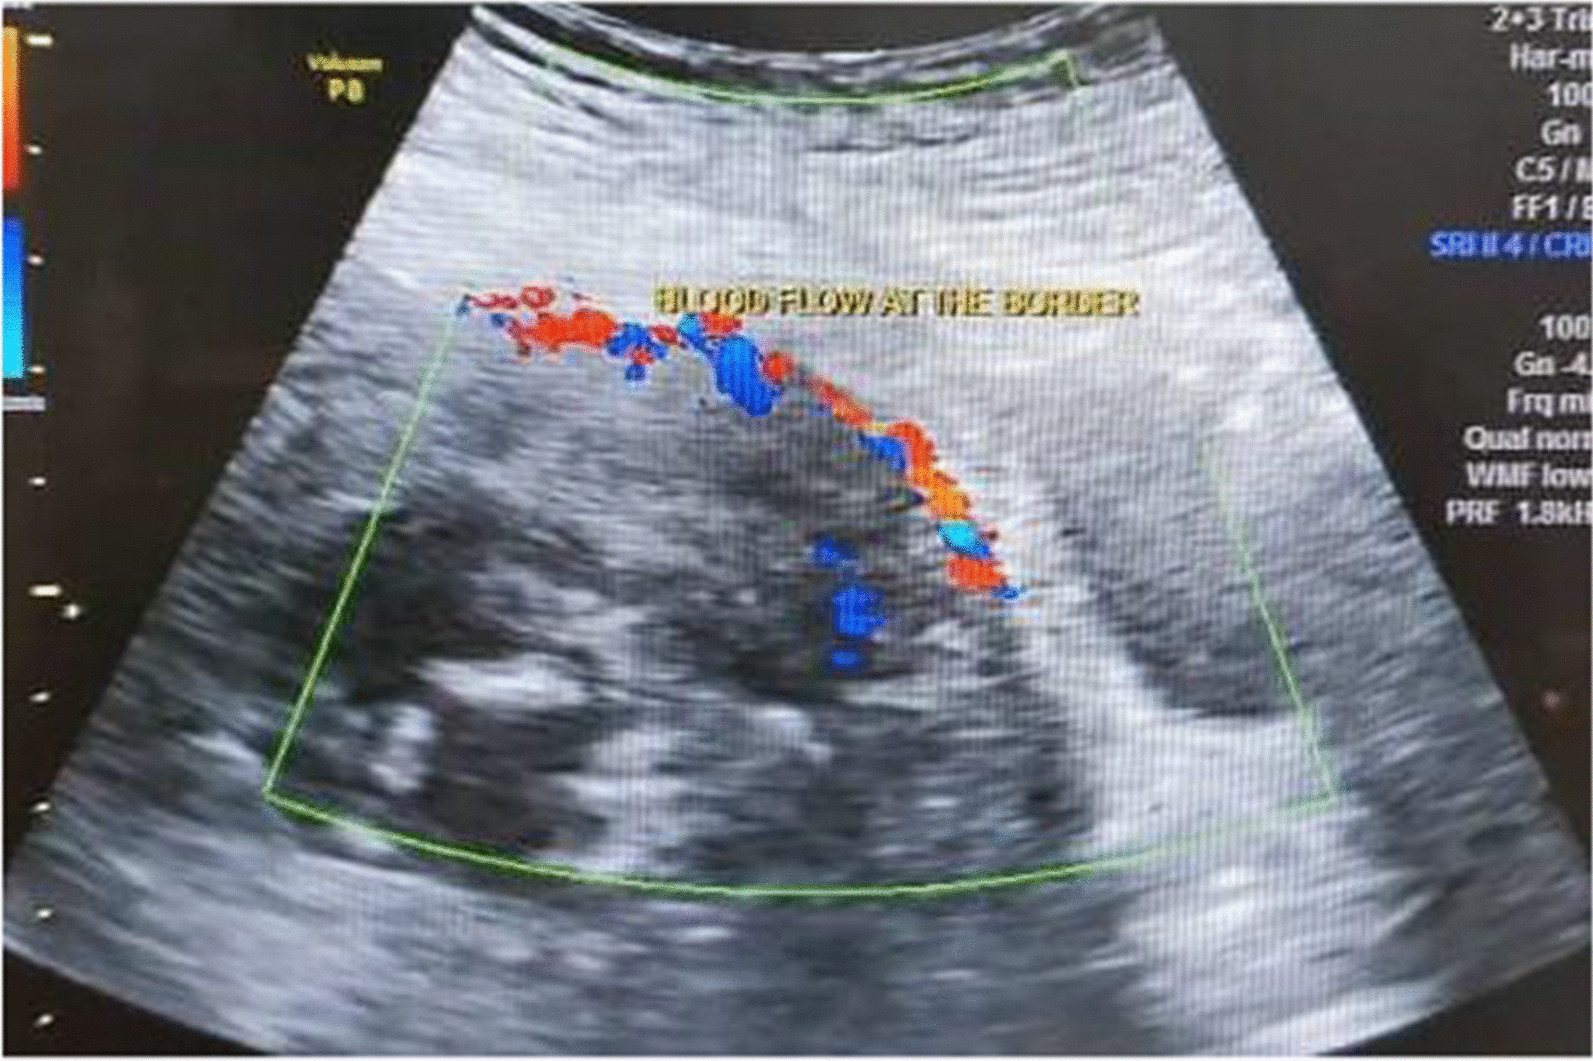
Transabdominal ultrasound showed an increase of blood flow between the uterus and urinary bladder border
